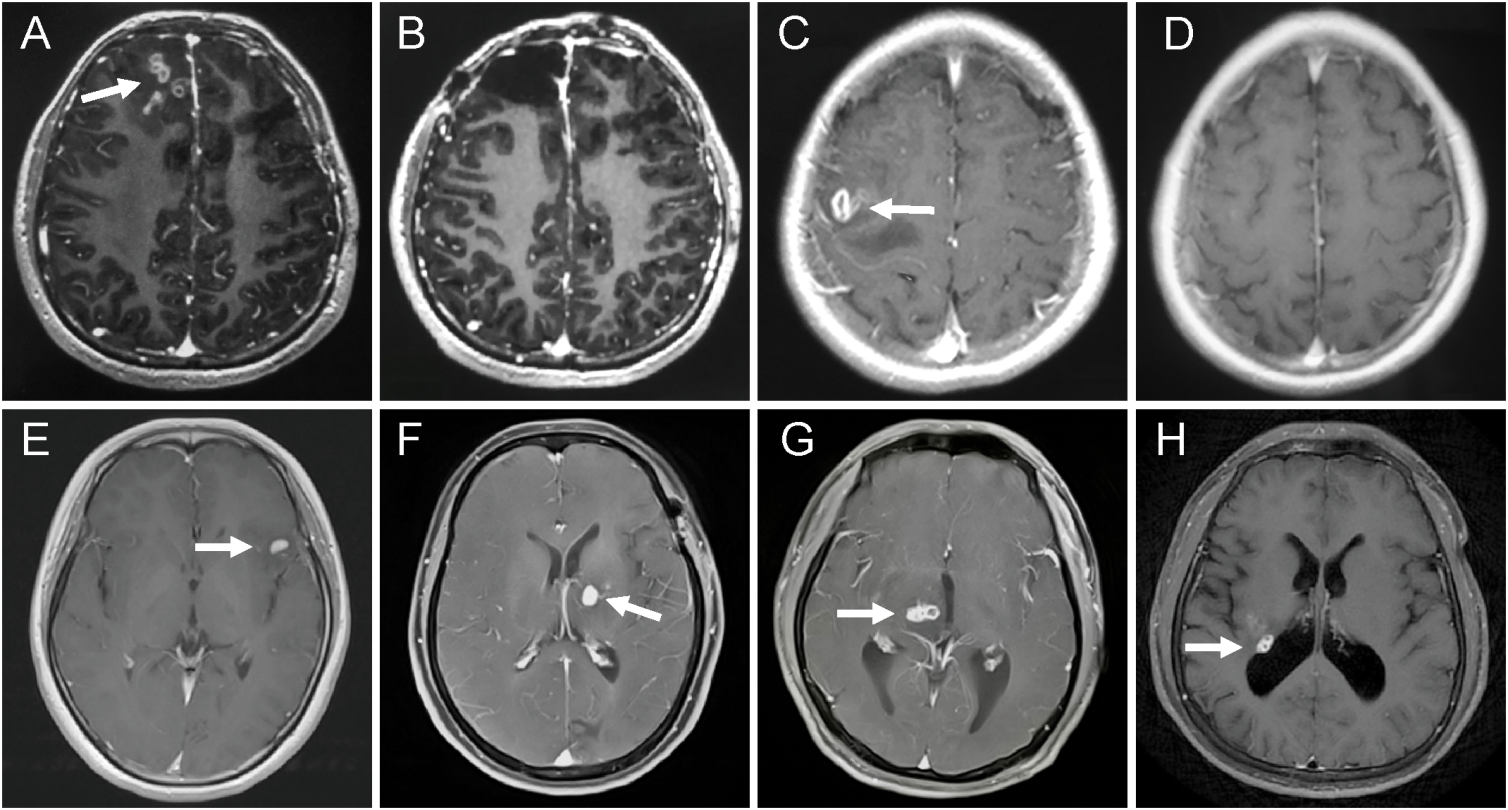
MRI changes of the patients with different treatment options and outcomes. (A–D) patients with good outcomes. (A) axial T1-weighted images revealed aggregated bead-shaped enhancement in the subcortical area of the right frontal lobe. (B) the active lesions were removed by surgery. (C) axial enhanced MRI showed nodular enhanced lesions in the left temporal lobe. (D) the enhanced lesions completely disappeared after 12 months with high-dose praziquantel. (E–H) patients with poor outcomes. (E) T1-weighted image demonstrating a crescent-shaped enhanced lesion in the left temporal lobe. (F) a new enhanced lesion reoccurred in the left basal ganglia at six months after surgery. (G) axial T1-weighted image showed a tunnel sign in the right thalamus. (H) T1-weighted image showed a round enhanced lesion in the right lateral posterior ventricle at 12 months after praziquantel treatment.
